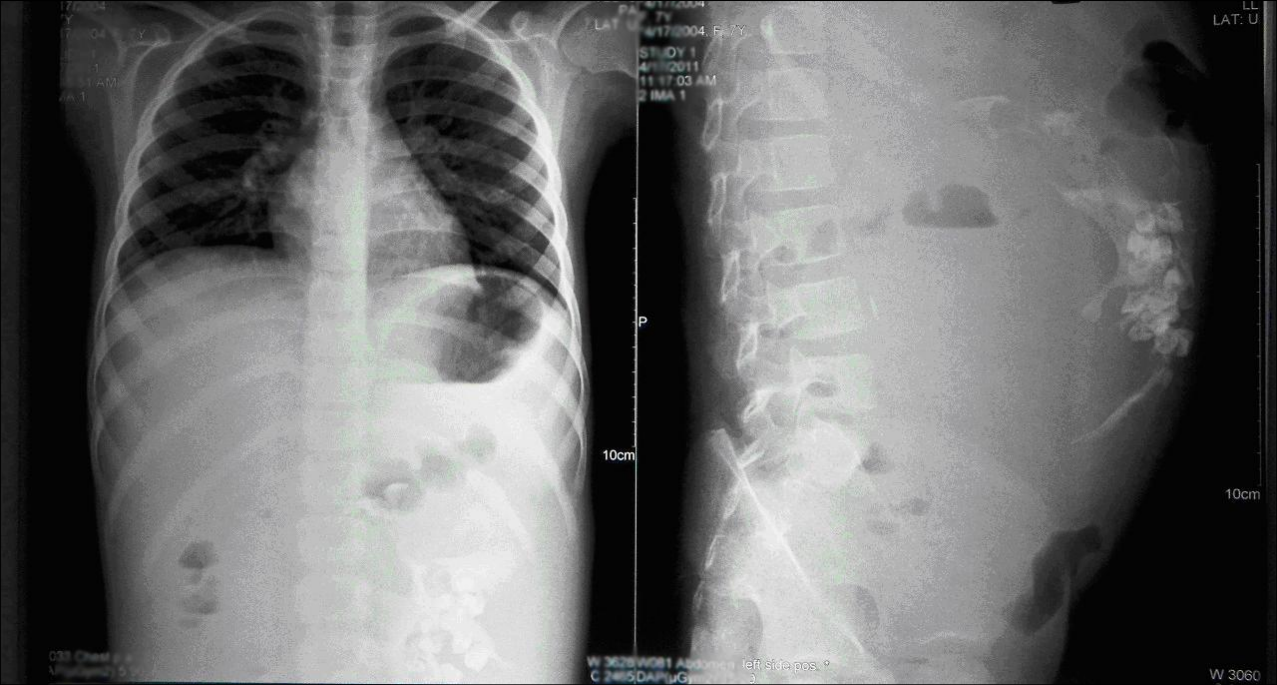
Figure 1: Abdominal roentgenograms showing soft-tissue mass displacing gut and having calcifications.
